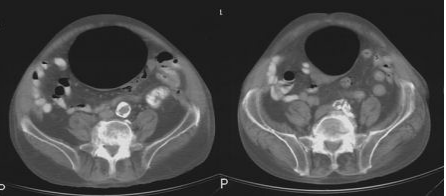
Selected axial sections from CT scan, demonstrating a large gas filled viscus.
